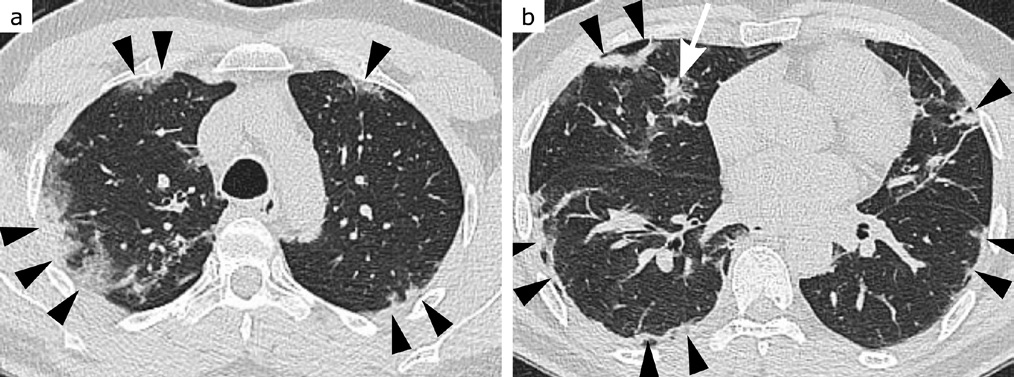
CO-RADS 5, consolidation type. 51-year-old patient with fever, cough and dyspnoea starting 8 days before the CT scan. Moderately elevated C-reactive protein and normal leukocyte levels. In upper lung zones (a) rounded, in lower lung zones (b) streaky consolidations in predominantly peripheral (arrowheads, a and b), but also central (arrow, b) location, indicating COVID-19 pneumonia. RT-PCR for SARS-CoV-2 returned positive
